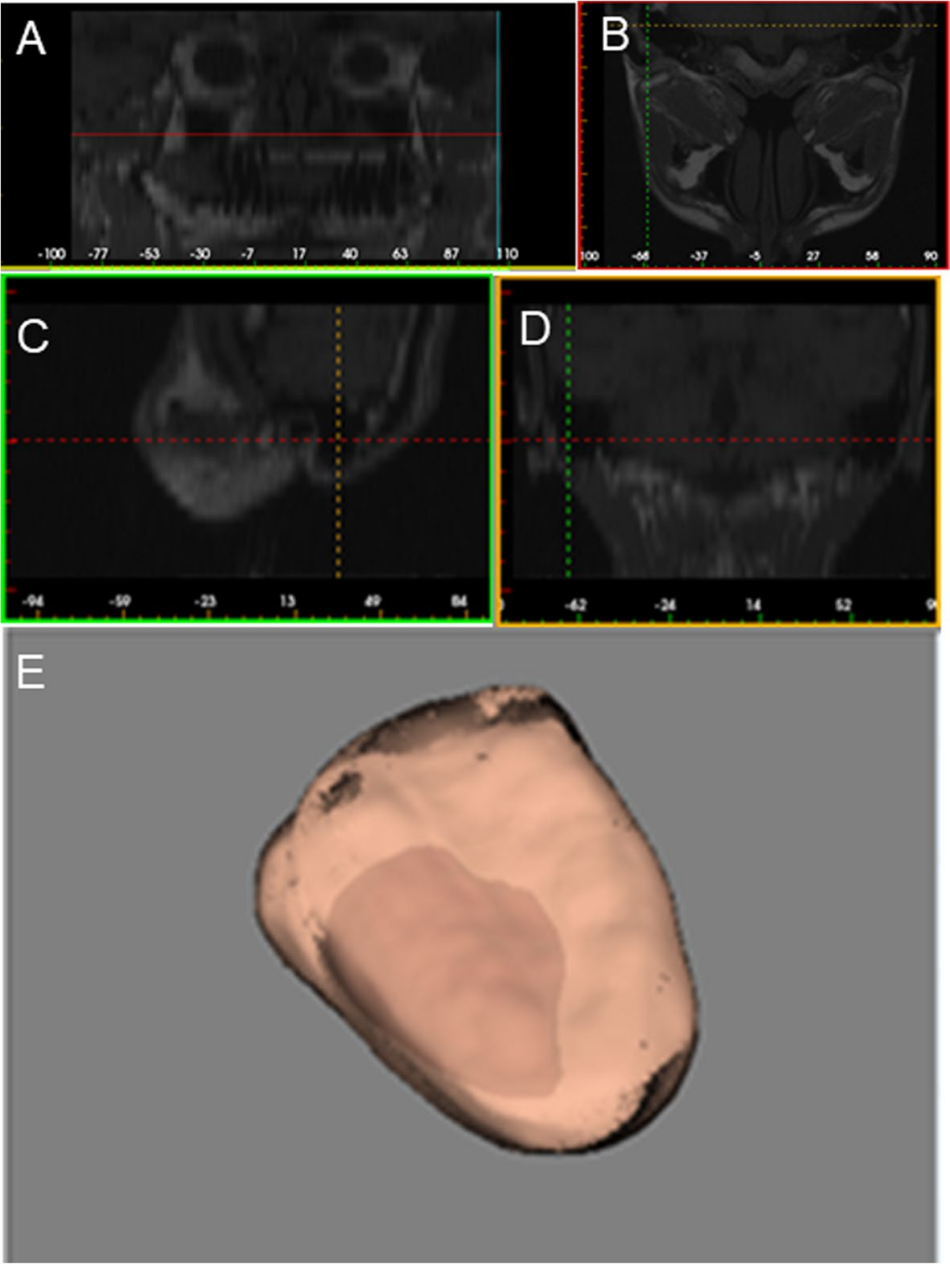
Generating a model of the tumor and the affected soft tissue. A Thin layer CT scan of curved planar reconstruction panorama. B Horizontal CT scan. C Sagittal CT scan. D Coronal CT scan. E 3D .stl model
